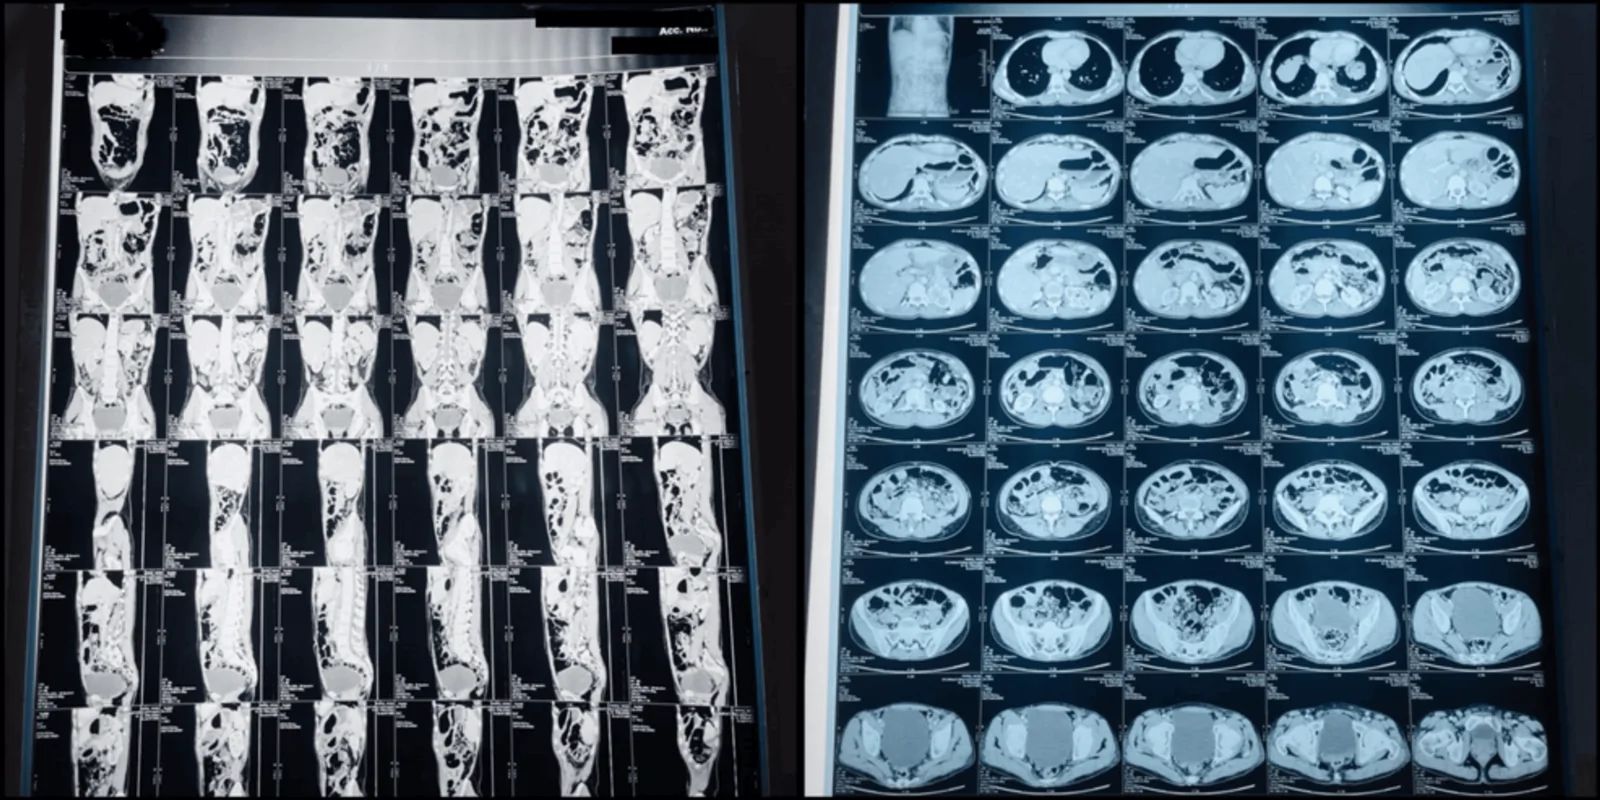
Contrast-enhanced computed tomography (CECT) of the whole abdomen showing multiple air foci in the peritoneal cavity (pneumoperitoneum), predominantly in the upper abdomen, with minimal to mild free fluid in the peritoneal cavity and minimal pleural effusion in the left thoracic cavity.
